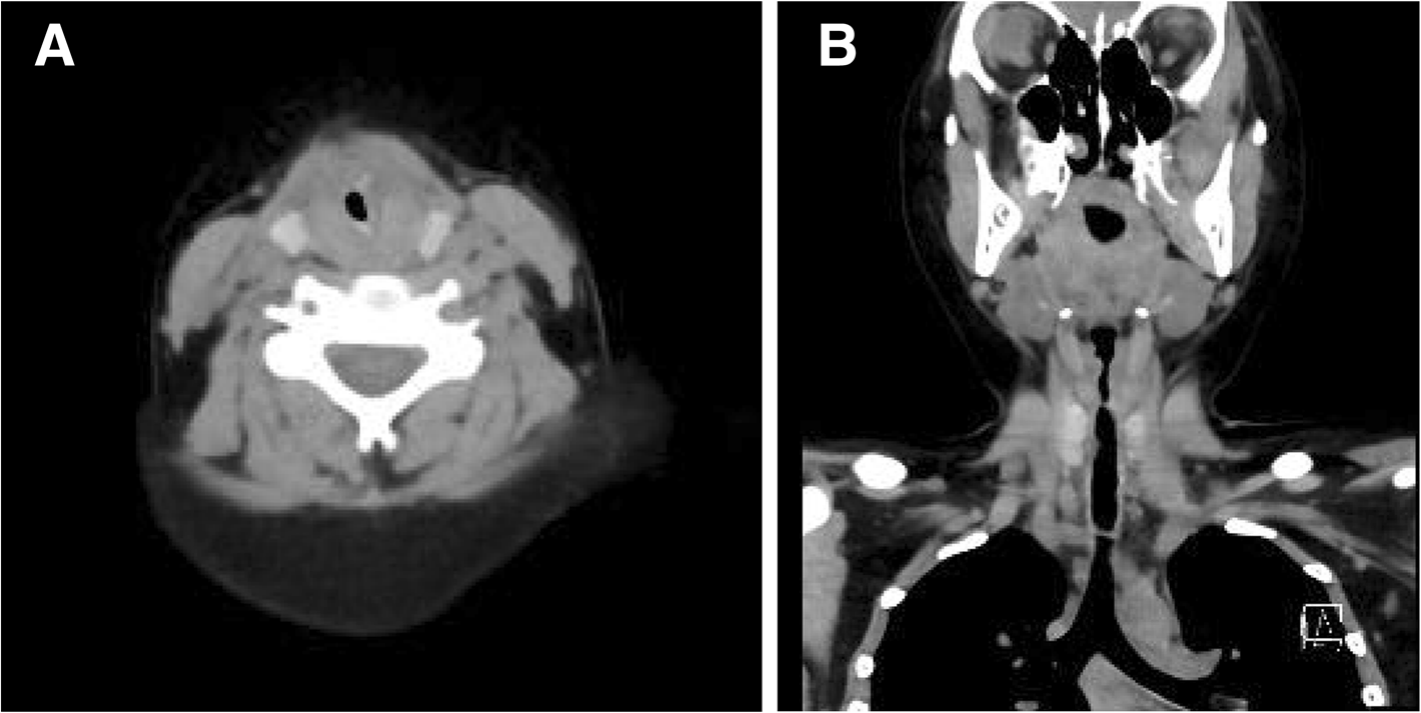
CT images of the neck with severe subglottic stenosis on admission
